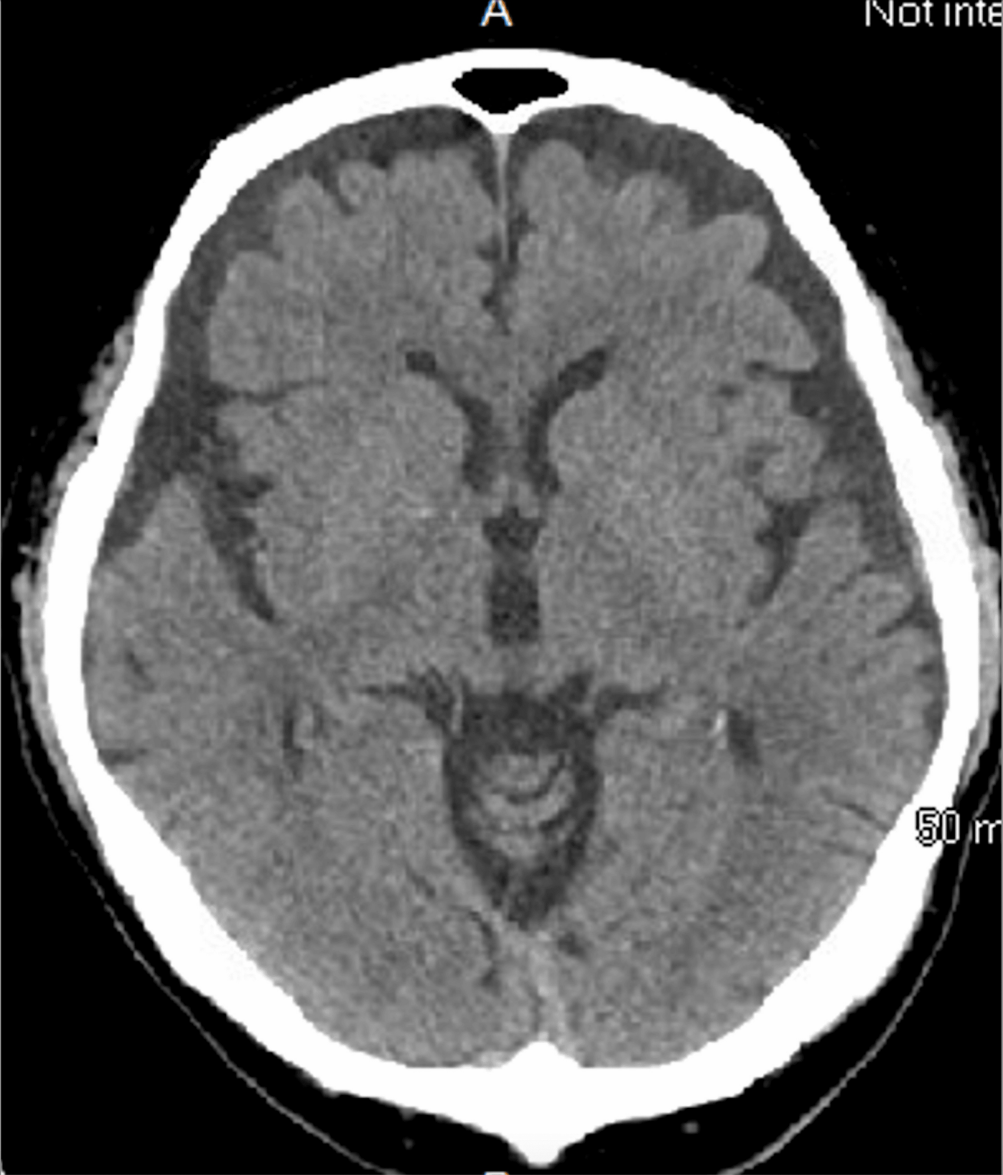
Non-contrast head CT scan showing no acute abnormalities
